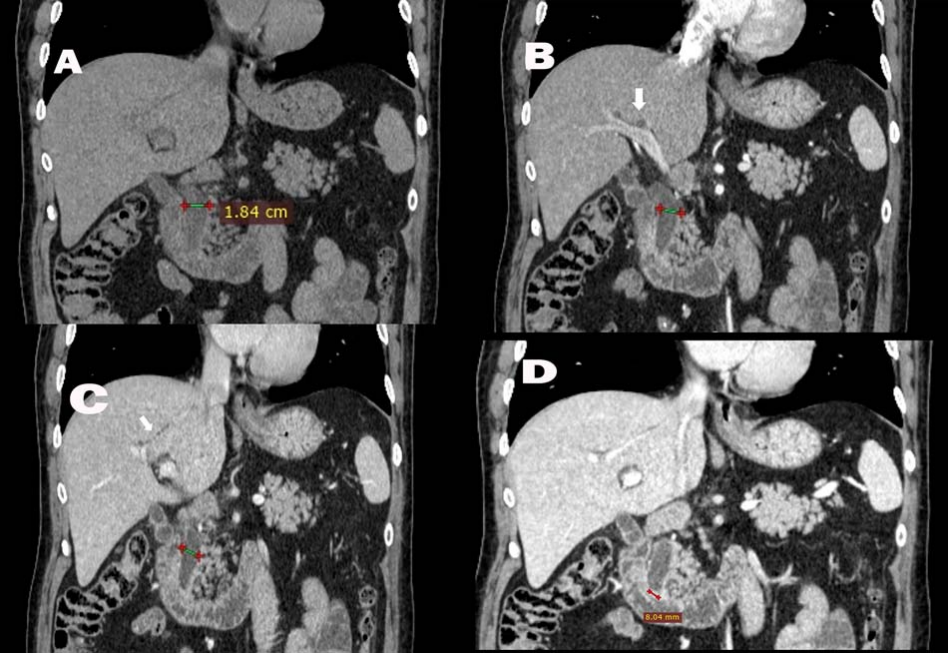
Quadruple-phase computed tomography. (A) Pre-contrast, (B) arterial phase and (C) venous phase Coronal CT (soft tissue window) have shown an intrahepatic (white arrow) and extrahepatic dilation of the biliary tree, with common bile duct (crosses) measuring 18.4 mm. No image of intrabiliary hyperdense/hypodense or contrast enhanced tissue was shown. (D) In the delayed phase of Coronal CT (soft tissue window), an enhanced tissue in the common bile duct, with a maximum diameter of 9 mm, was identified.
